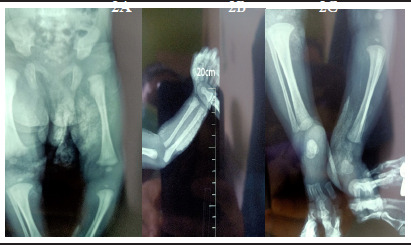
Image 2A: X-ray image showing soft tissue calcification over the thighs, Image 2B: X-ray showing linear calcification over the forearm, Image 2C: X-ray image showing calcification over the ankle
